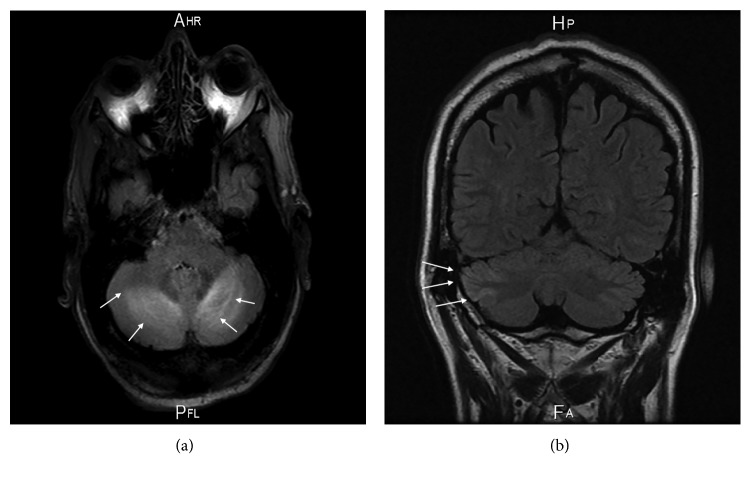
Cranial MRI displays high signal intensity in both cerebellar hemispheres six months prior to admission (a); and, currently, a residual hyperintensity in the right cerebellar hemisphere on T2-weighted and FLAIR images (b).
